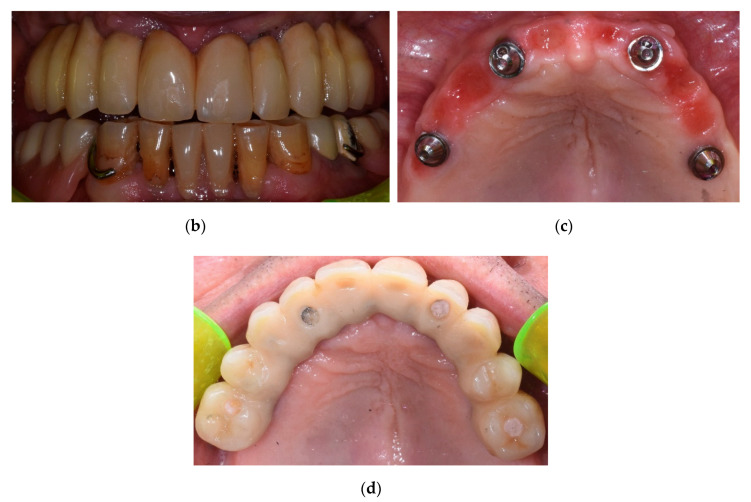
Panoramic radiograph of a full-arch immediate loading rehabilitation of the upper jaw according to the Columbus Bridge Protocol (a); frontal view (b) and occlusal intraoral views of the patient with (d) and without (c) prosthesis.
